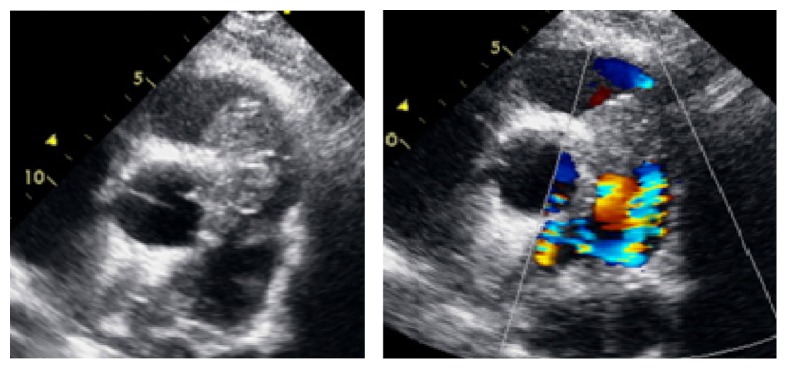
2D transthoracic echo images in short axis parasternal view showing a large echodense RVOT mass, with signal aliasing on color Doppler.
